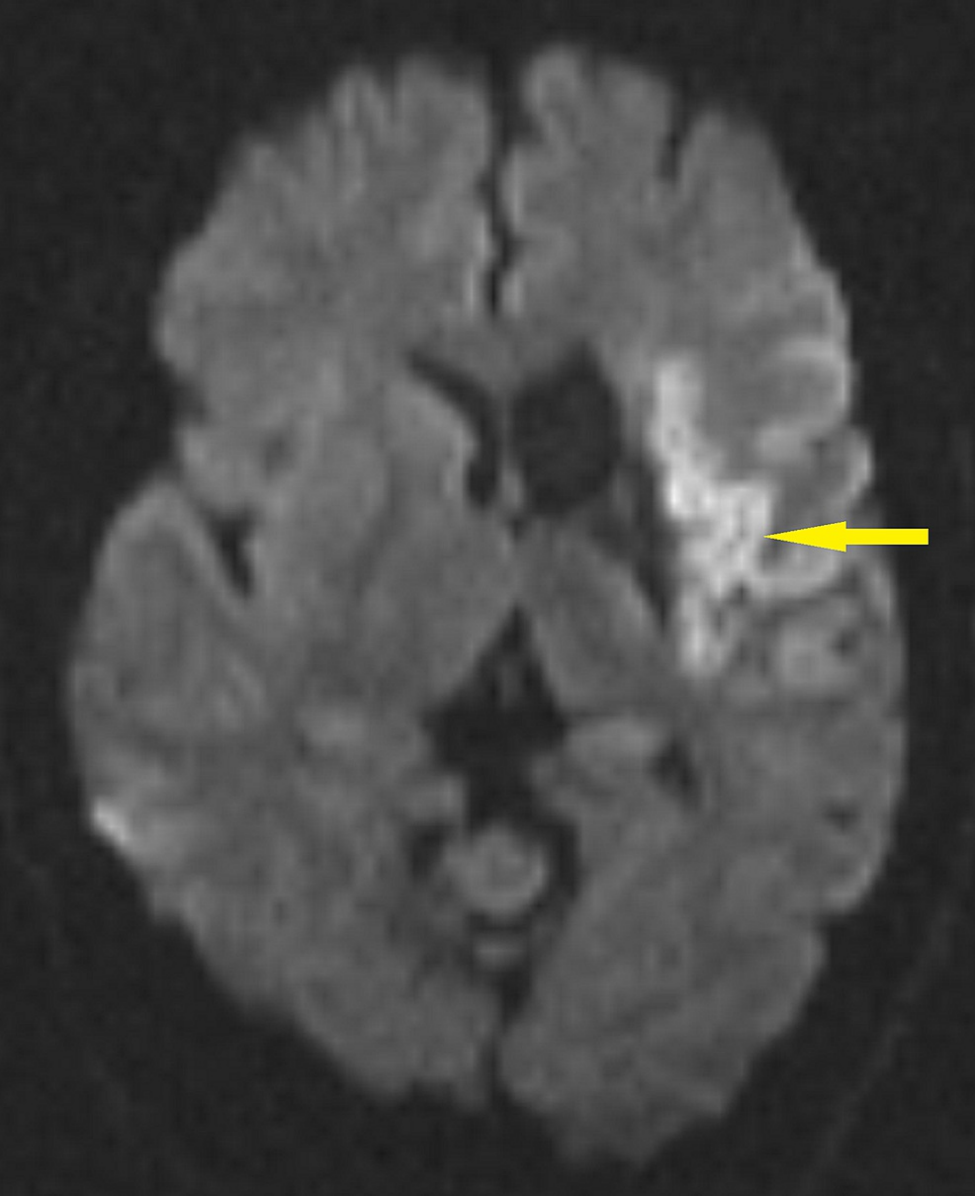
MRI brain axial DWI showing large acute infarct involving the left frontal lobe and left insular cortex (07/2019) DWI, Diffusion Weighted Imaging
